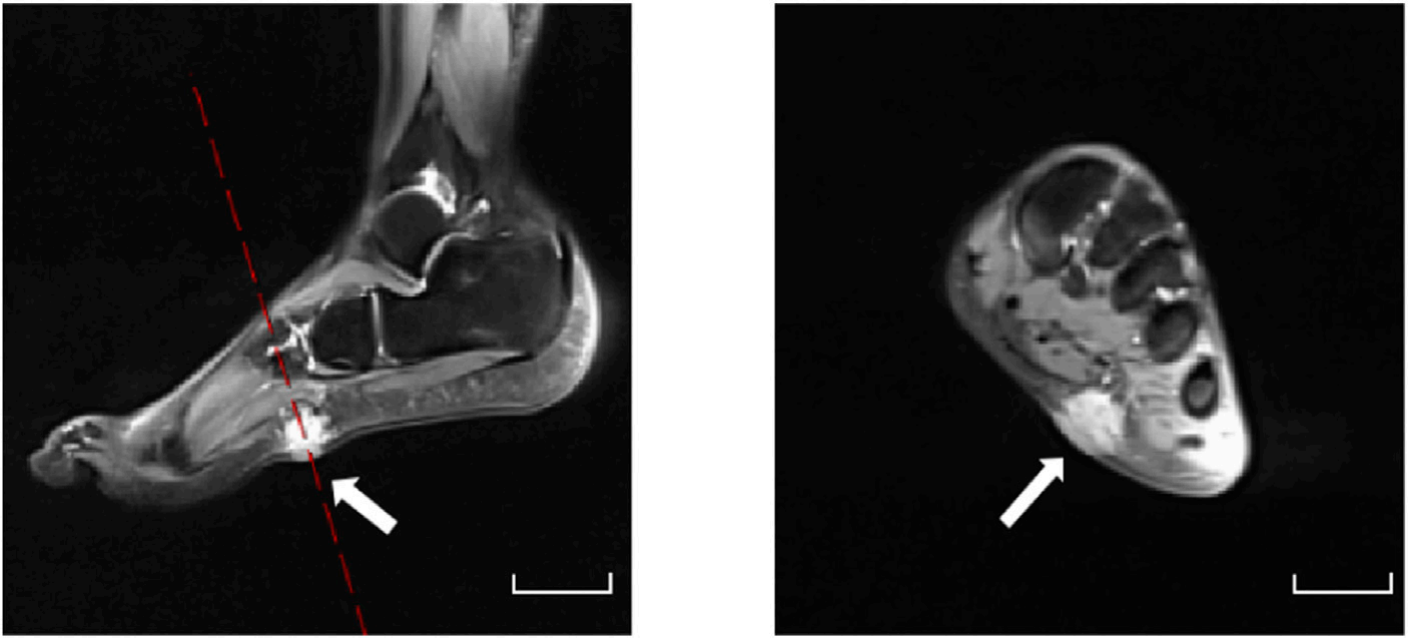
Plantar MRI. Pre-treatment MRI of the left plantar region showing a lobulated subcutaneous lesion (4 × 3 cm) along the lateral plantar aspect, isointense on T1-WI and hyperintense on fat-suppressed T2-WI. Scale bars, 5 cm.
